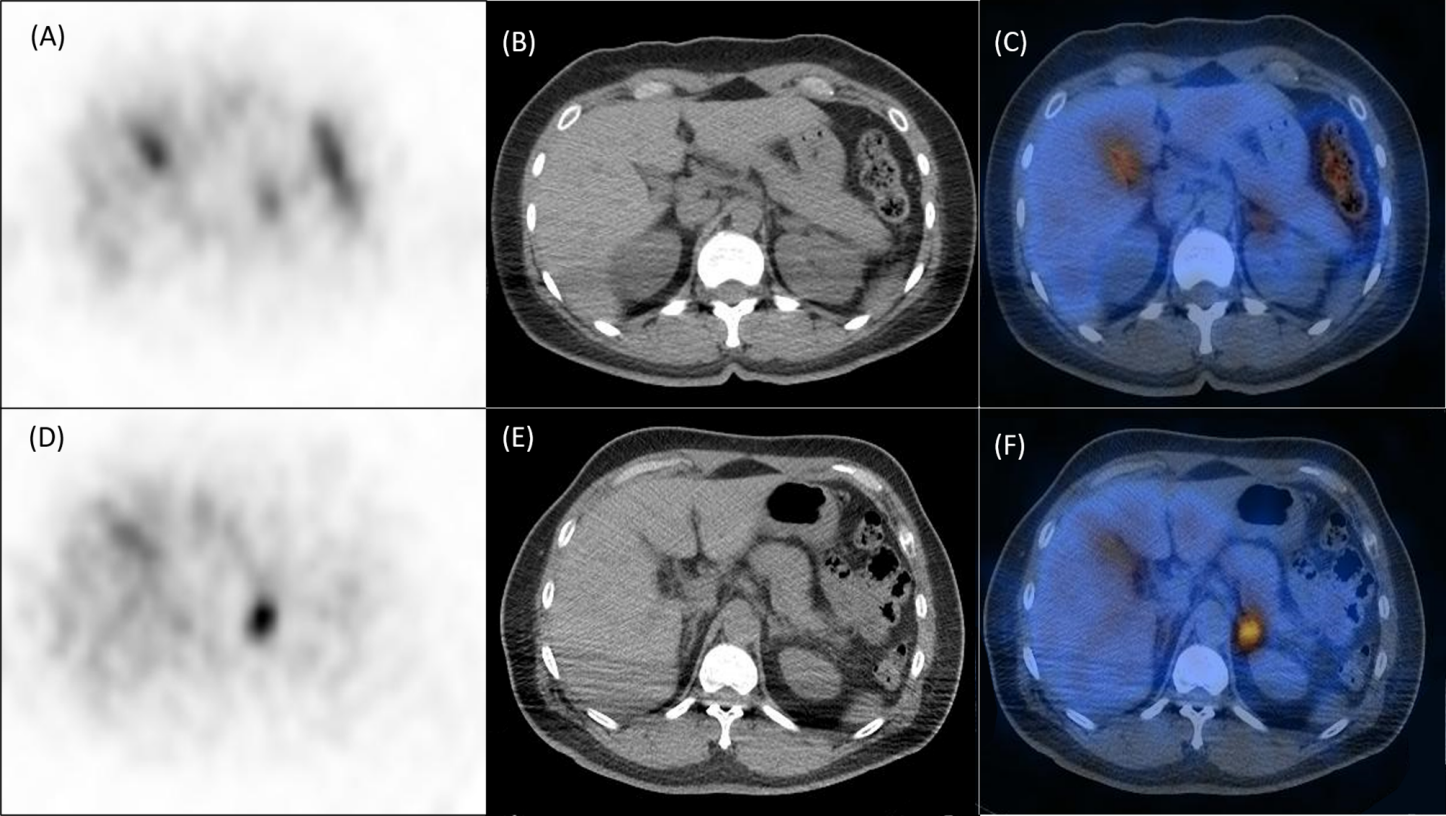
Representative NP-59 adrenal SPECT/CT and *KCNJ5* mutation status. Upper panel, findings of a 40-year-old female without *KCNJ5* mutation. SPECT (A), CT (B) and fusion SPECT/CT (C) showed moderate NP-59 uptake at left adrenal gland with ALR of 2.62 and CON of 1.38. Lower panel, findings of a 54-year-old male with *KCNJ5* mutation. SPECT (D), CT (E) and fusion SPECT/CT (F) showed significant NP-59 uptake at left adrenal gland with ALR of 3.72 and CON of 2.41.
